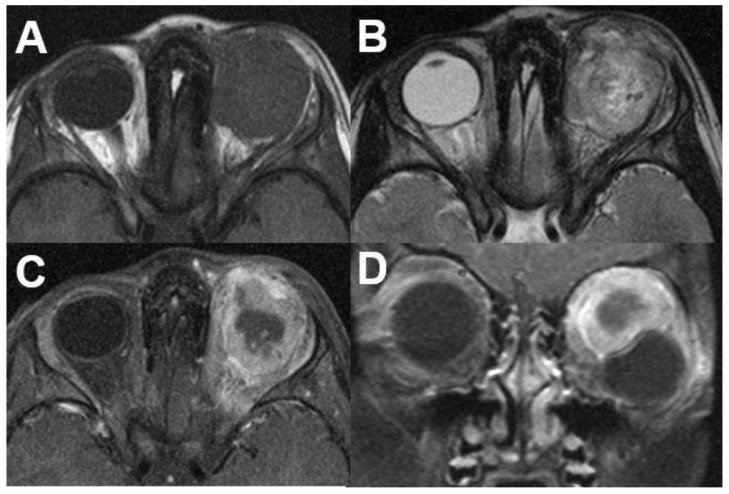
Rhabdomyosarcoma (RMS).* MRI of a 4-year-old female patient with RMS of the left-sided orbit. The tumor shows hypointense signal on T1-weighted images (A) and inhomogeneous hyperintense signal on T2-weighted images (B). The center of the lesion shows marked hyperintensity compared to the contralateral side. Peripheral enhancement and central absent enhancement is seen after contrast administration, matching necrotic portions (C). The bulb is compressed and displaced caudally (D).
